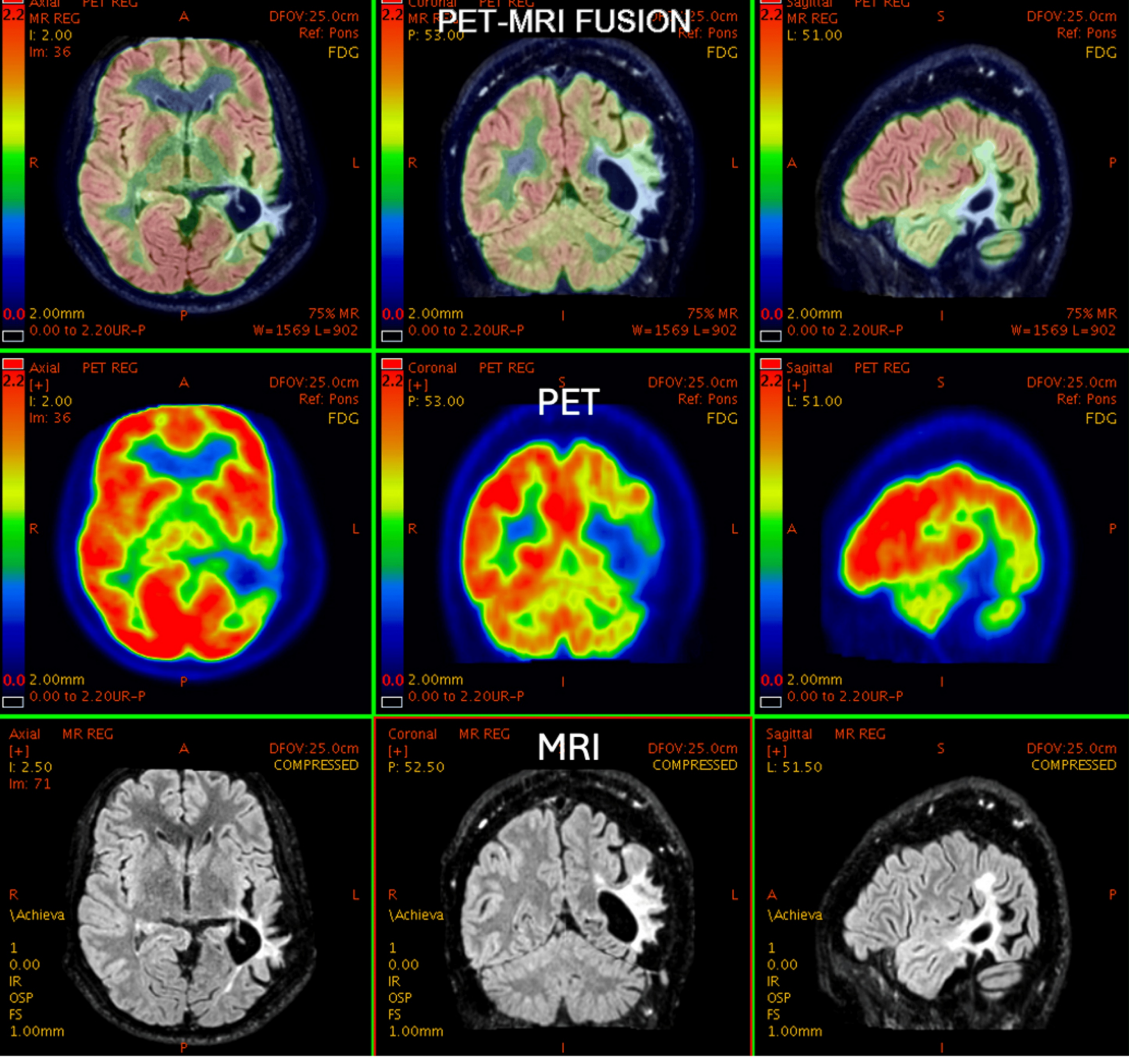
25-year-old male with a history of seizure disorder. PET-MRI brain study shows a gliotic area involving left parietal region, left superior temporal gyrus, and left middle temporal gyrus with prominence of overlying sulcal spaces underlying left temporal horn - left lateral ventricle confluence. Corresponding areas of PET images show decreased FDG uptake compared to the opposite side, suggesting decreased metabolic activity secondary to gliosis and volume loss. PET: positron emission tomography; MRI: magnetic resonance imaging; FDG: fluorodeoxyglucose
